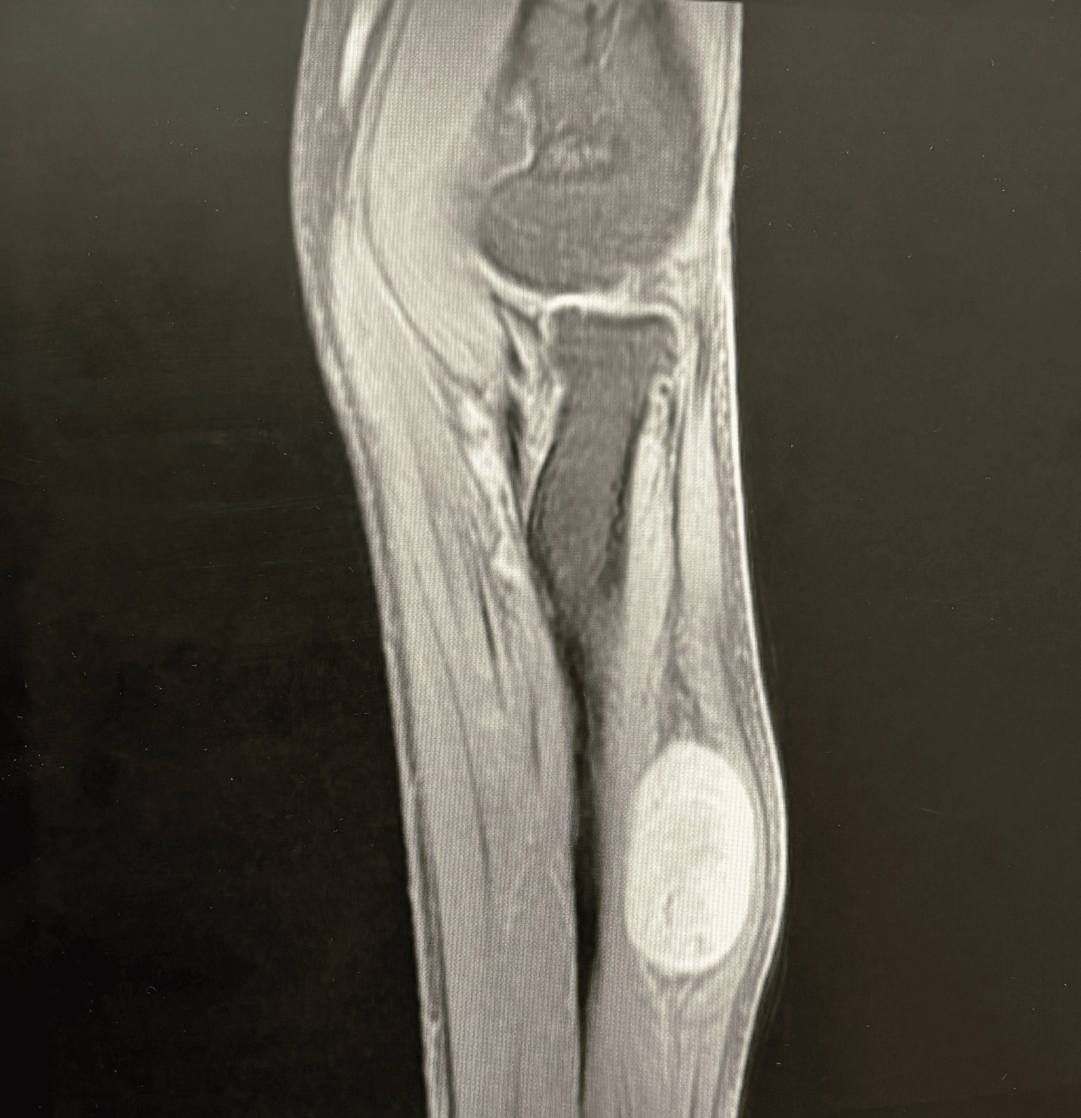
MRI sagital section showing well-defined PIN schwannoma MRI: magnetic resonance imaging; PIN: posterior interosseous nerve
